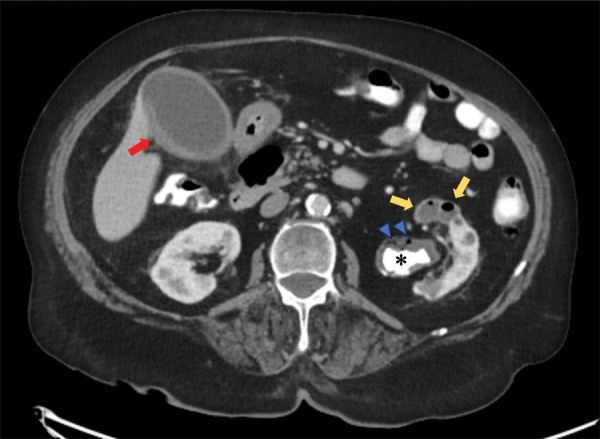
CT abdomen - axial plane.
